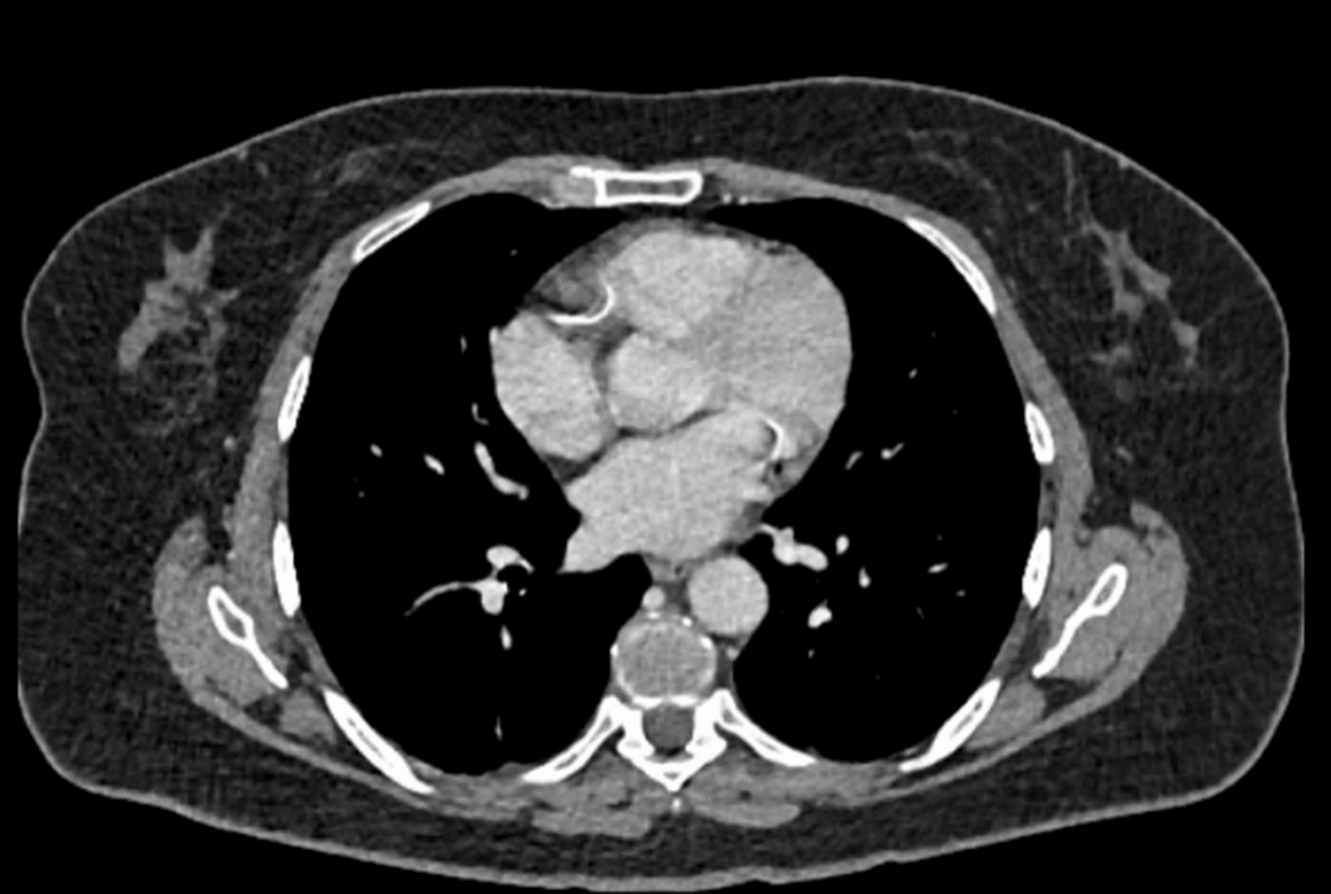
Axial computed tomography (CT) scan of the thorax demonstrating no evidence of metastatic disease.
